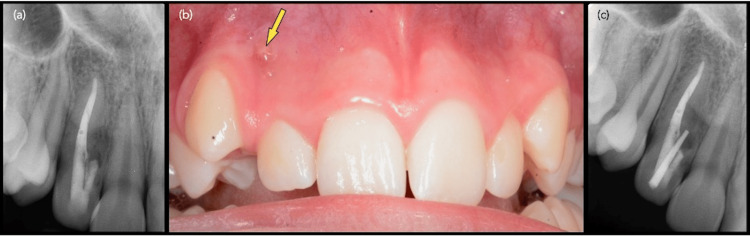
(a) Twelve-month periapical radiograph showing a persistent mid-radicular radiolucency. (b) Intraoral photograph at 12-month recall after nonsurgical root canal treatment, revealing an active draining sinus tract on the mid-buccal plate between teeth #12 and #13 (arrow) (×6.3). (c) Radiograph with gutta-percha point tracing the sinus tract toward the mesial radiolucent area.
